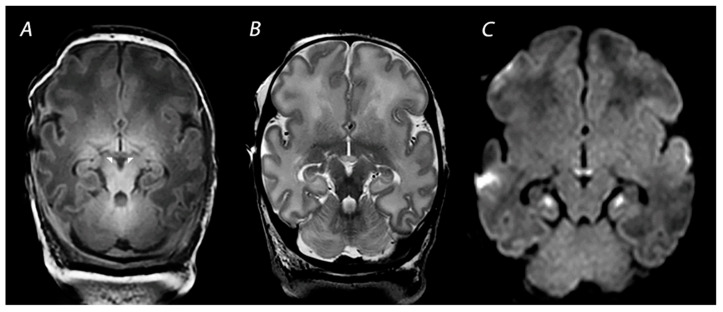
MRI performed on 10 days after birth showing injury of the mammillary bodies, presenting as decreased signal intensity on T1 weighted imaging (indicated by the white arrows on (A), swelling and increased signal intensity on T2-weighted imaging (B), and diffusion restriction on diffusion-weighted imaging (C). Diffusion restriction is also noted in the hippocampi, which is often seen in combination with mammillary body injury.
